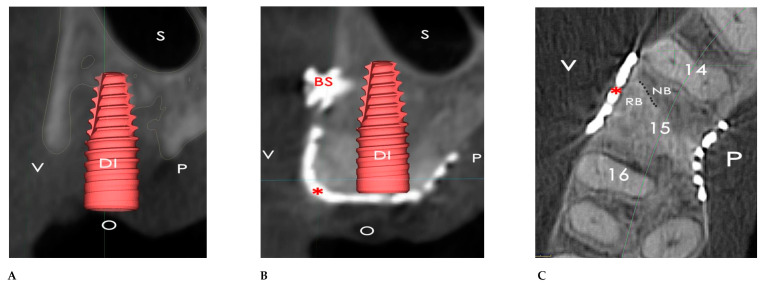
(A) Vestibule–palatal section of the baseline CBCT. The dental implant (DI) was almost uncovered by bone. (B) Vestibule–palatal section of 6-month secondary CBCT. A regular-size 10 mm dental implant (DI) was inserted in place of tooth 15. The titanium mesh (*) was integrated with the new-formed alveolar bone, without any black zone between the mesh and the regenerated bone. BS: bone screw; V: vestibulum; P: palate; O: occlusal; S: maxillary sinus. (C) Transversal section of the 6-month follow-up CBCT showed the integration between the native alveolar bone (NB) and the regenerated bone (RB), which is outlined with a dotted line. *: titanium mesh; V: vestibulum; P: palate.
